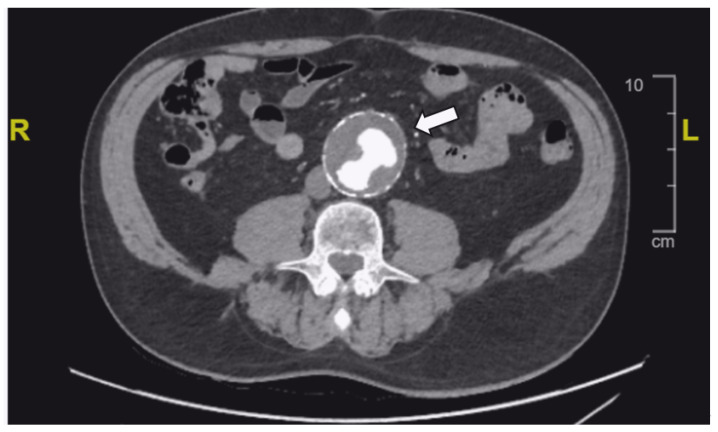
The CT scan demonstrating the large abdominal aortic aneurysm (arrow).
